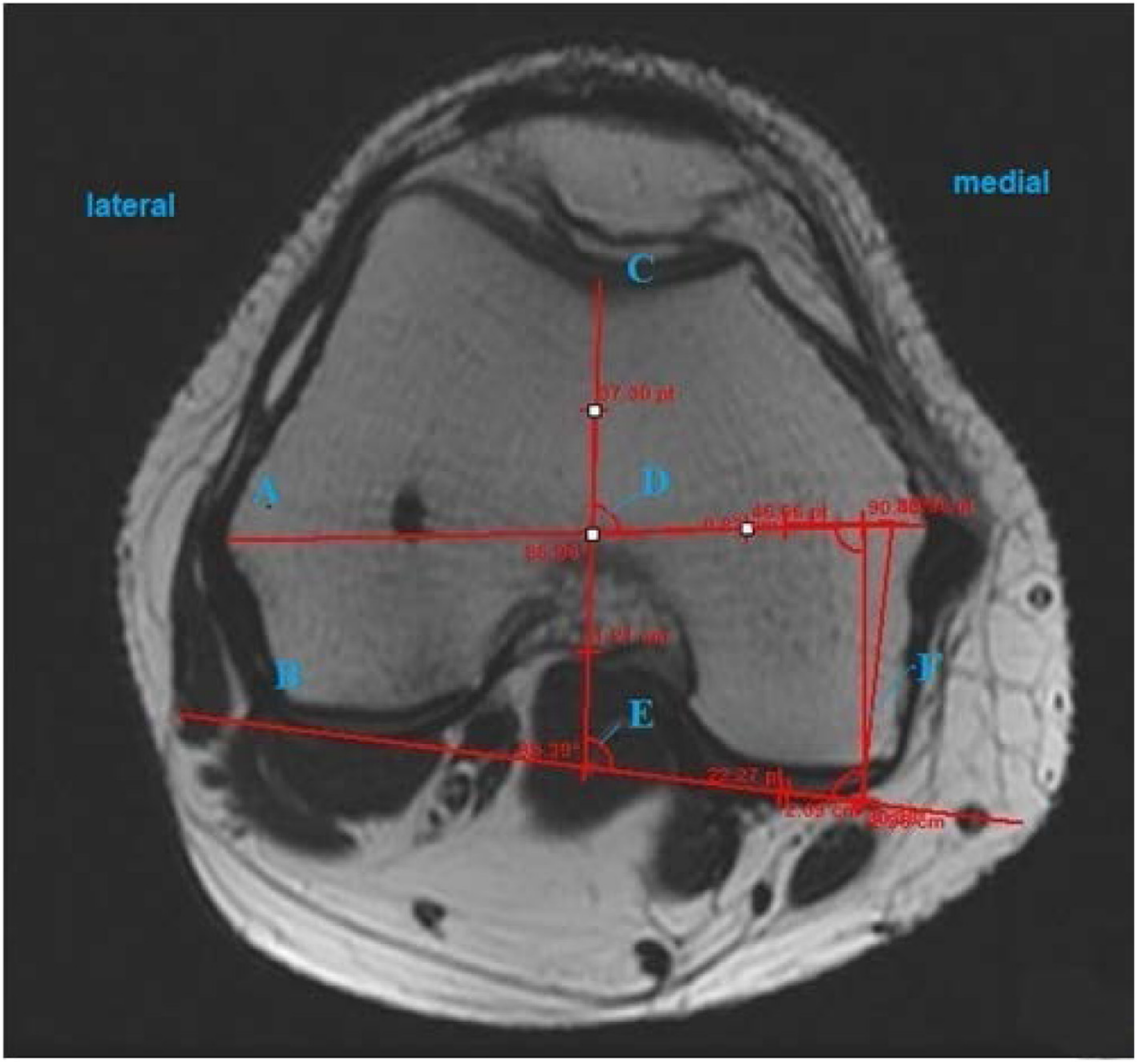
MRI of the distal femur with the axes and angles. *A* Clinical epicondylar axis, *B* posterior condylar axis, *C* anteroposterior axis, *D* Whiteside-epicondylar angle, *E* Whiteside-posterior condylar angle, *F* condylar twist angle
